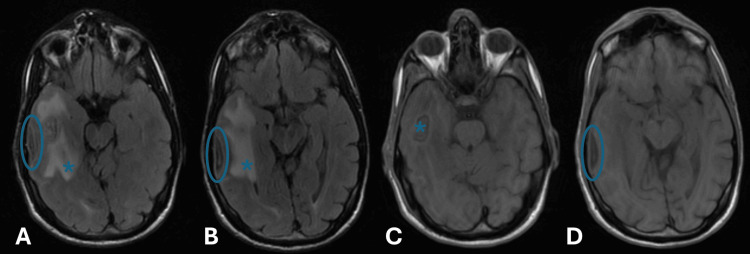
Brain MRI after one week of treatment showing an increase in abscess (*) and subdural empyema (blue circle). A and B are axial T2 flair slices; C and D are axial T1 slices. MRI: magnetic resonance imaging
